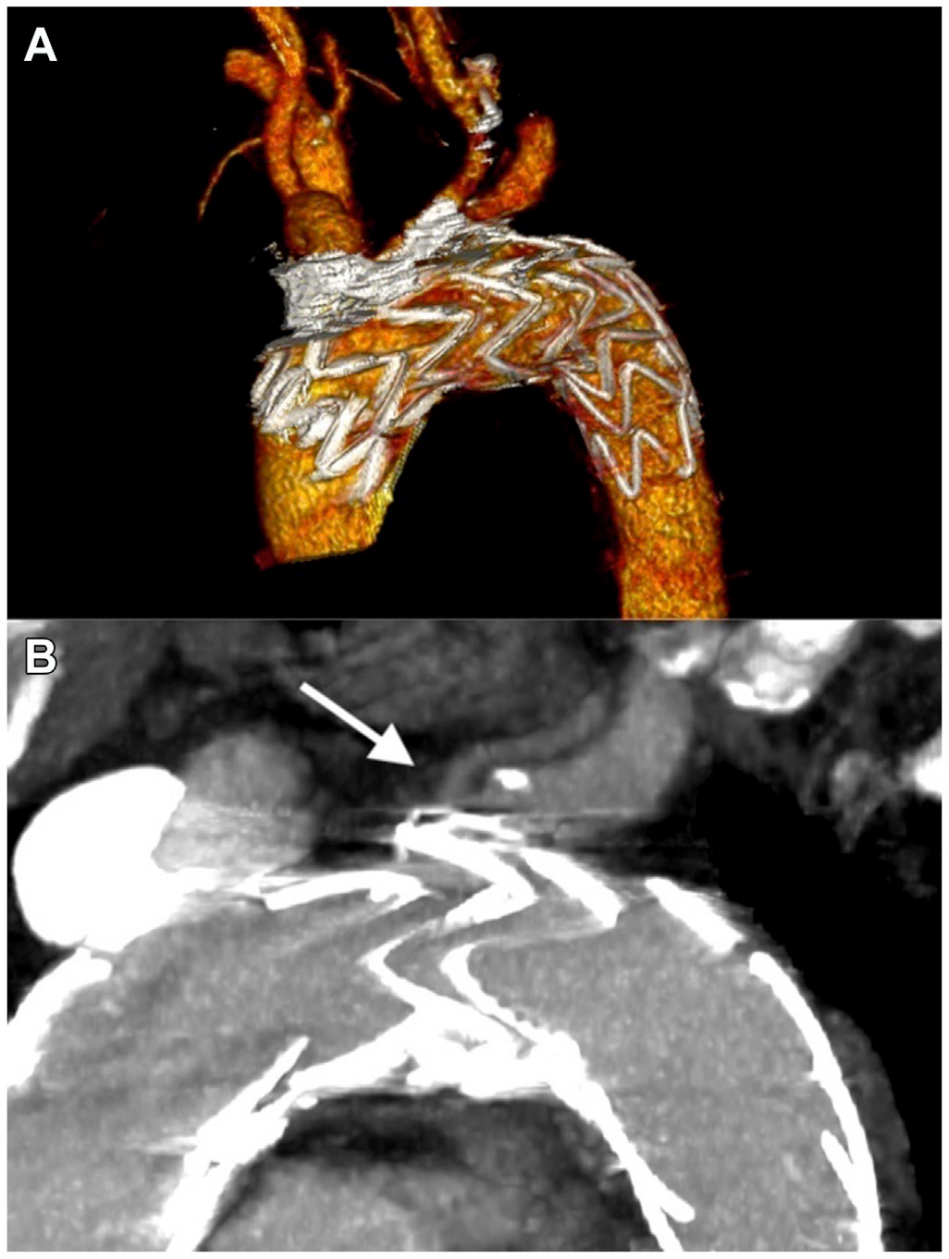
(A) No leaks nor other complications were detected at the postoperative computed tomography angiography (CTA). (B) The white arrow indicates good patency of the left vertebral artery (LVA).
